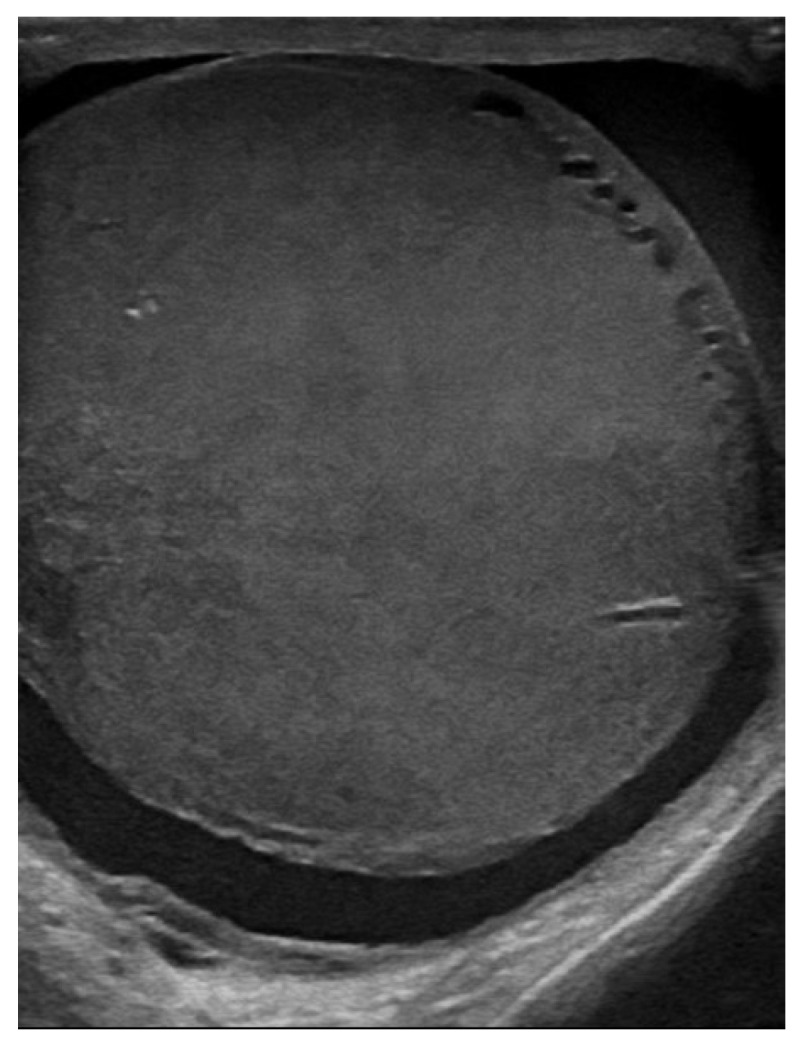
Axial ultrasound section of the left testicle: increased size, inhomogeneous echostructure, and alternating hypoechoic areas.
